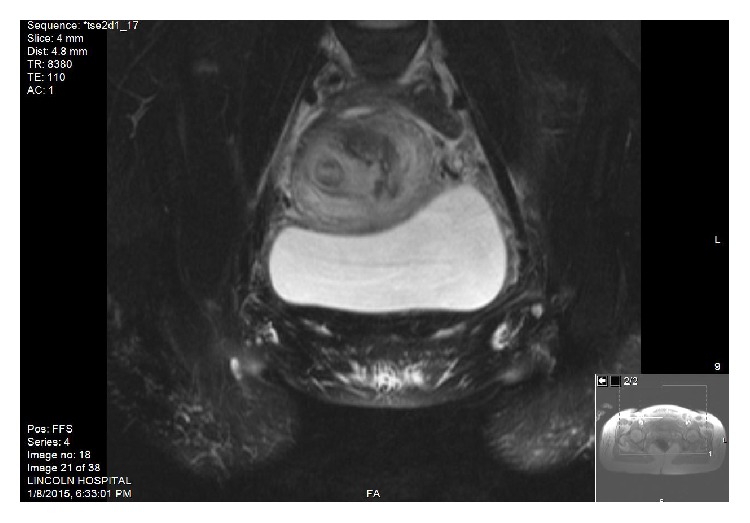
MRI of abdomen and pelvis with contrast: Series # 4 coronal FS (Fast): right uterus and left bulky postpartum uterus.
